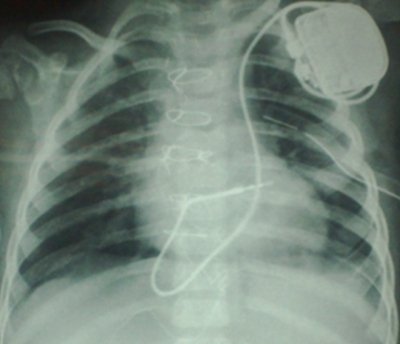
Chest X-ray shows endocardial pacemaker implantation via the persistent left superior vena cava and coronary sinus. Note that the active ventricular lead is fixed into the high interventricular septum
